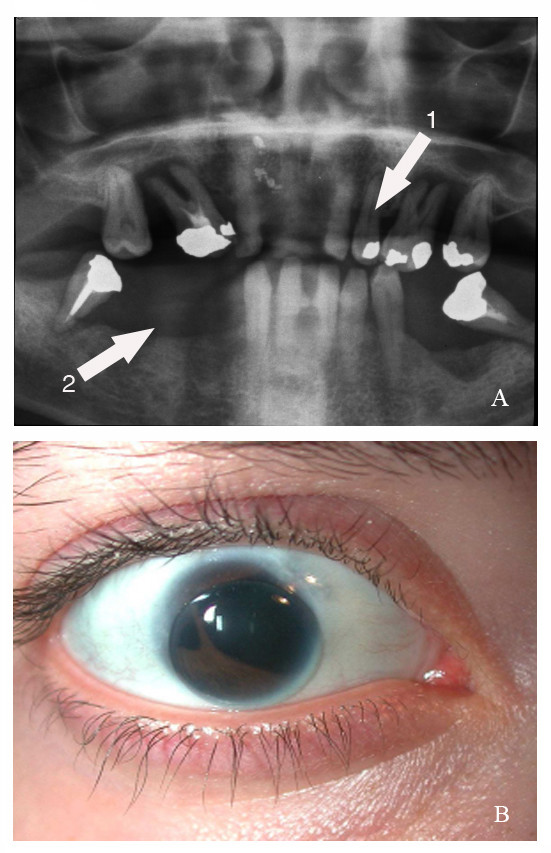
Phenotypic analysis of patient II-1. A) Panoramic dental X-ray showing hypodontia (arrow 1) and area of maxillary hypoplasia (arrow 2), B: Eye imaging showing polycoria.
